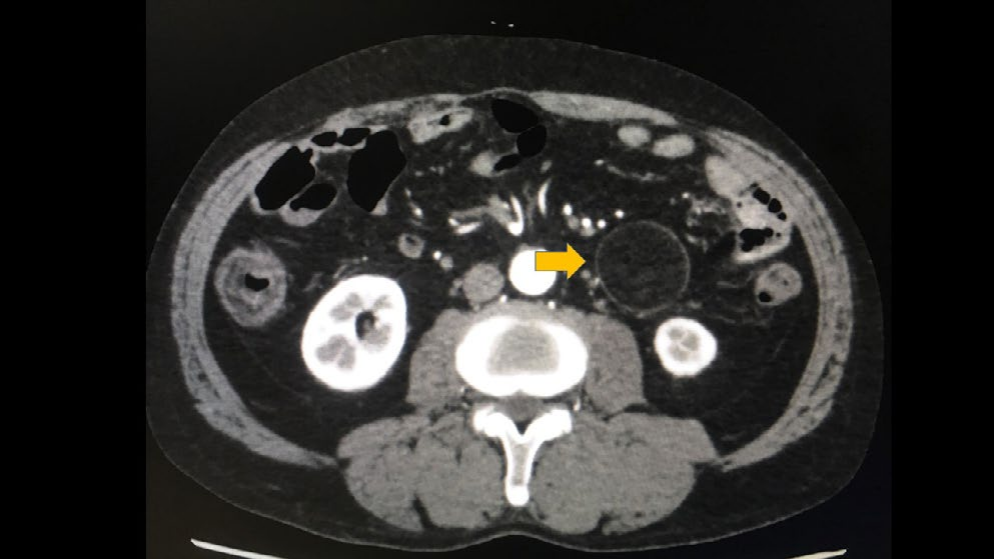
Computed tomographic image of the abdomen, demonstrating a round and encapsulated 4 × 4 cm mass without any contrast enhancement
